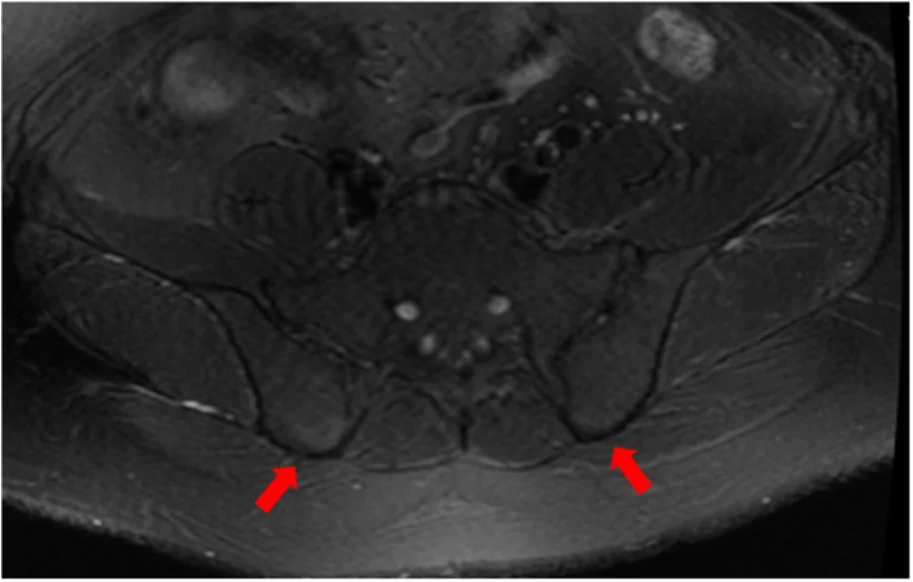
MRI imaging demonstrated bilateral sacroiliitis. (arrows).
